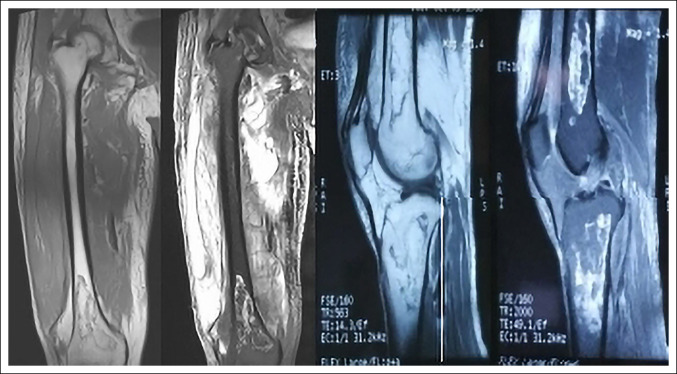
MRI showing early bone infarction in the distal femur and proximal tibia with local soft-tissue abscess in the right thigh, high signal on T1WI, and mixed signal on T2WI.
